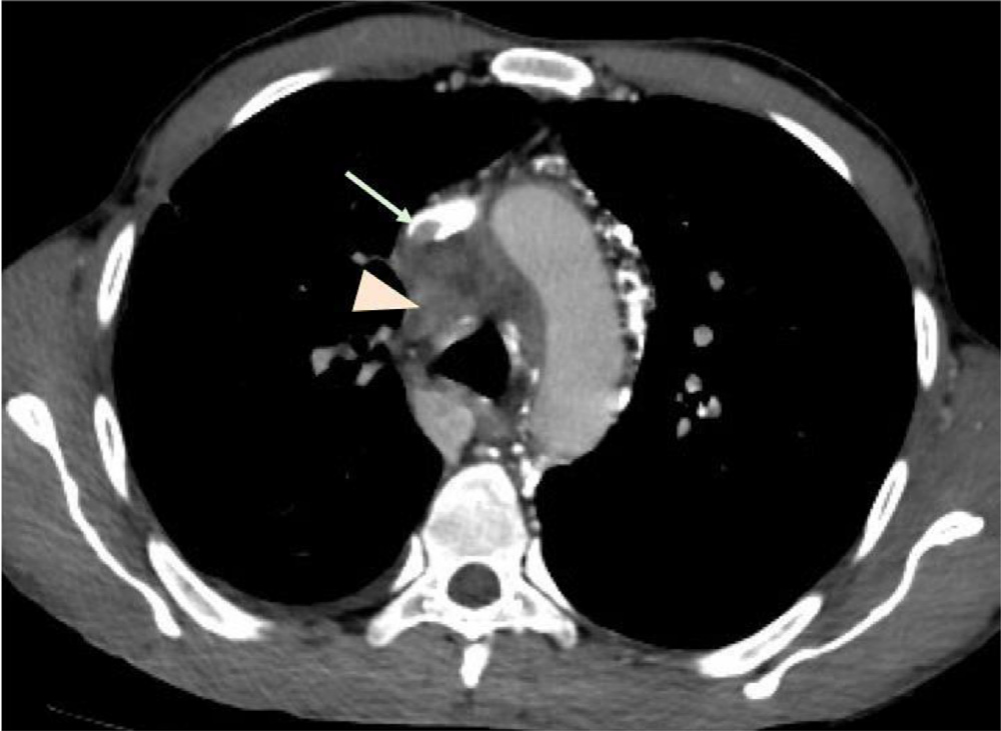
Axial contrast-enhanced CT image (soft-tissue window) showing infiltrative heterogeneous soft tissue (Arrowhead) encasing superior vena cava which is thrombosed (Arrow). We note also para-aortic collateral channels due to vena cava obliteration.
